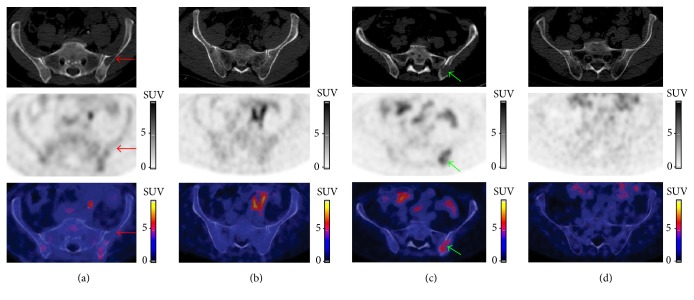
[^18^F]FPRGD_2_ PET/CT images of all patients. A diffuse bone marrow involvement was described based on CT images in patients #1 (a) and #2 (b); the [^18^F]FPRGD_2_ PET images also showed diffuse and heterogeneous bone marrow [^18^F]FPRGD_2_ uptake in patients #1 (a) and a mild diffuse bone marrow uptake in patient #2 (b). In contrast, no diffuse bone marrow [^18^F]FPRGD_2_ uptake was seen in patients #3 ((c) the green arrows point at a lytic FL with [^18^F]FPRGD_2_ uptake) and #4 ((d) no lesion shown). Note that, in patient #1 (a) with newly diagnosed MM, a large osteolytic FL in CT images did not show [^18^F]FPRGD_2_ uptake above the bone marrow background ((a) red arrows).
